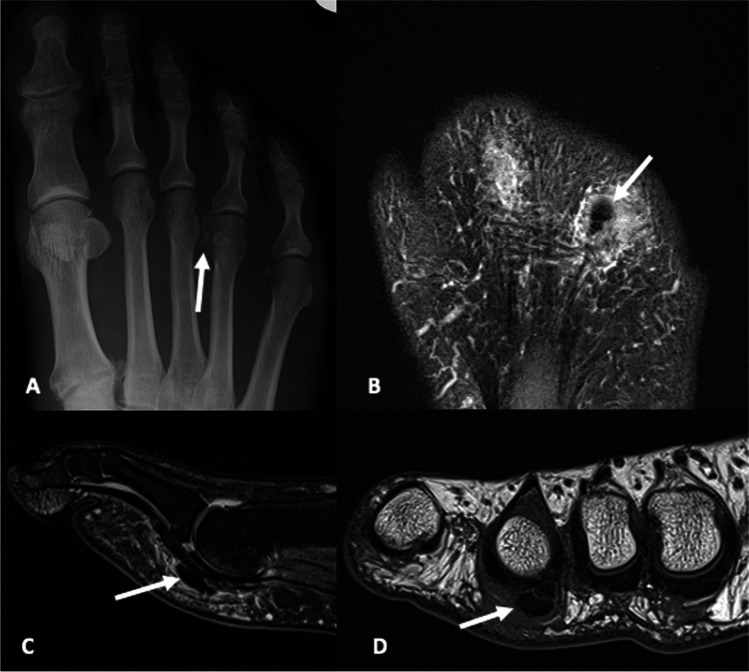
A 48-year-old male presented with pain at the plantar aspect of the right 4th metatarsophalangeal joint. A AP radiograph of the foot demonstrates two foci of calcification adjacent to the medial aspect of the 4th MTP joint (arrow). B Long axis proton density with fat saturation, C sagittal proton density with fat saturation and D short axis T1 MRI images demonstrate two foci of calcification involving the plantar/medial capsule of the 4th MTP joint (arrow) with adjacent soft tissue edema
